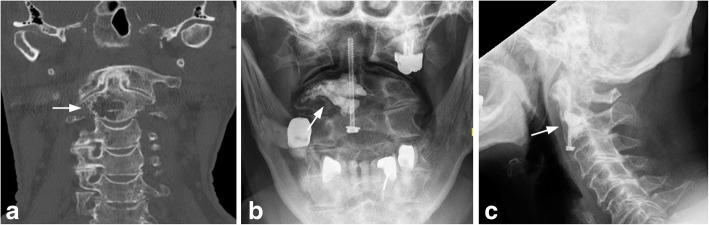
CT-scan of the upper cervical spine in lateral view with osteolytic lesion of C2 (arrow) of patient with vertebral hemangioma (a). Postoperative x-rays show a good filling of osteolytic lesion and the additional anterior odontoid screw (b and c) (Case 4)
